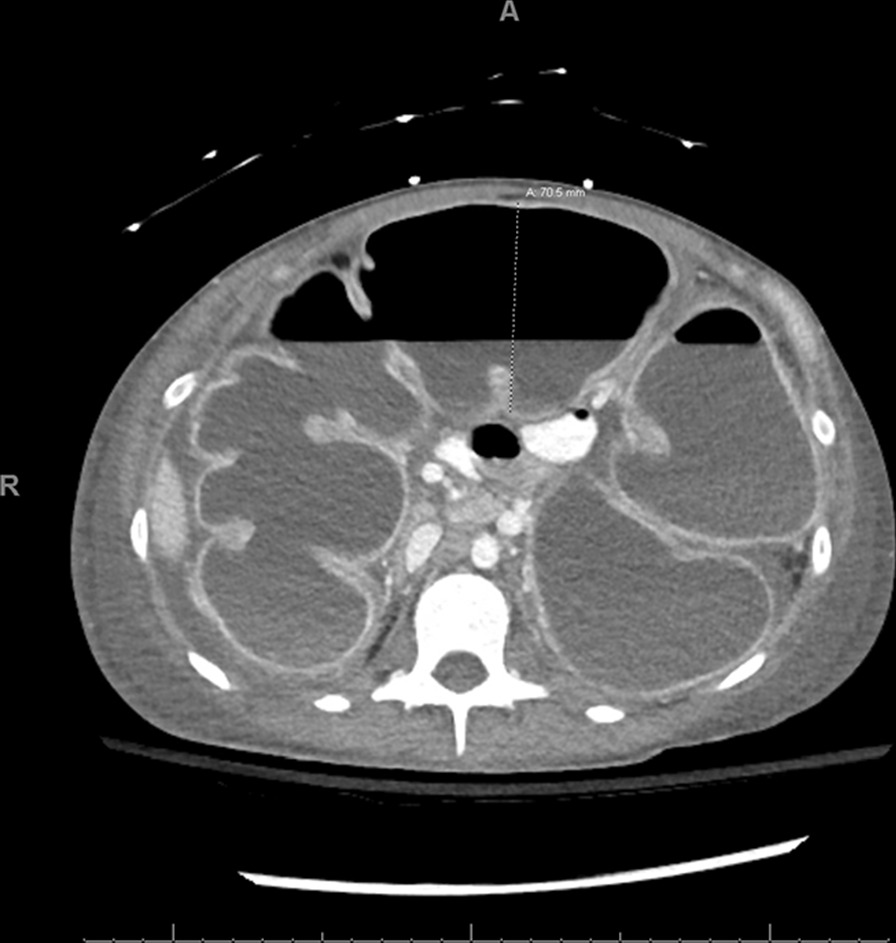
Axial slice of a computed tomography abdomen/pelvis showing dilated large bowel with partial enhancement of the wall of the colon, suggestive of colitis
